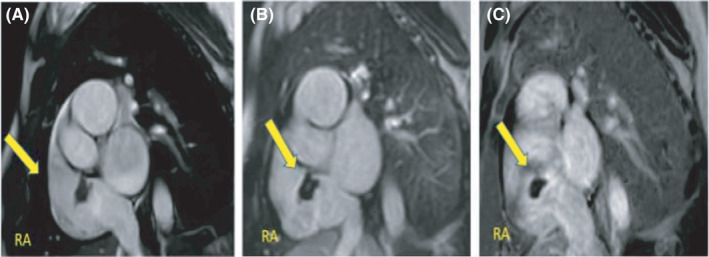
Cardiac MRI atrial short axis planes A, precontrast short axis SSFP CINE still image showing hypointense lobulated structure (yellow arrow) inferior to the SVC, and post gadolinium early B, and delayed enhancement C, short axis views showing no contrast uptake within the right atrial structure, consistent with atrial thrombus
